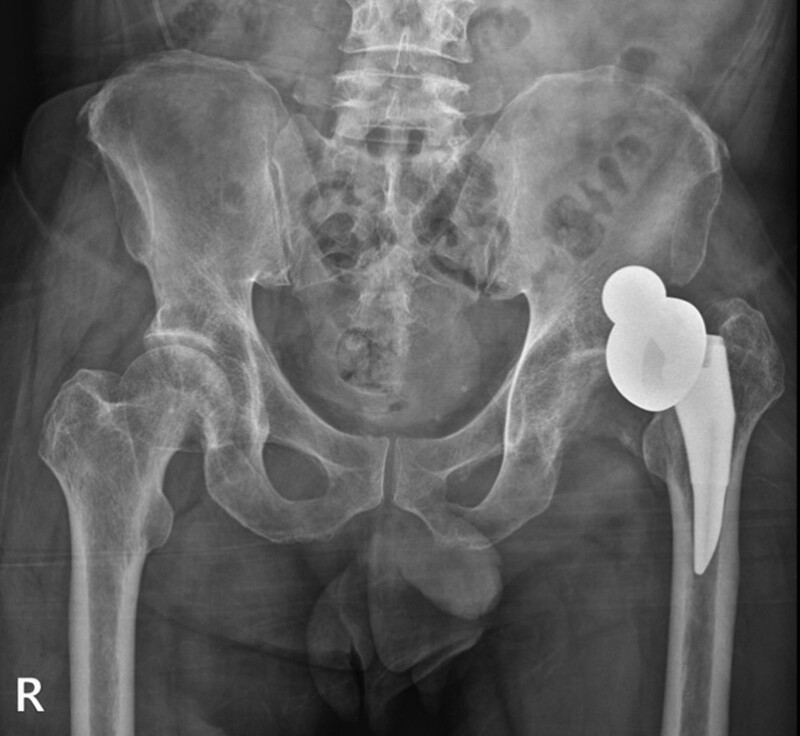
Anteroposterior radiograph showing the dissociation between bipolar cup components following manual reduction.
